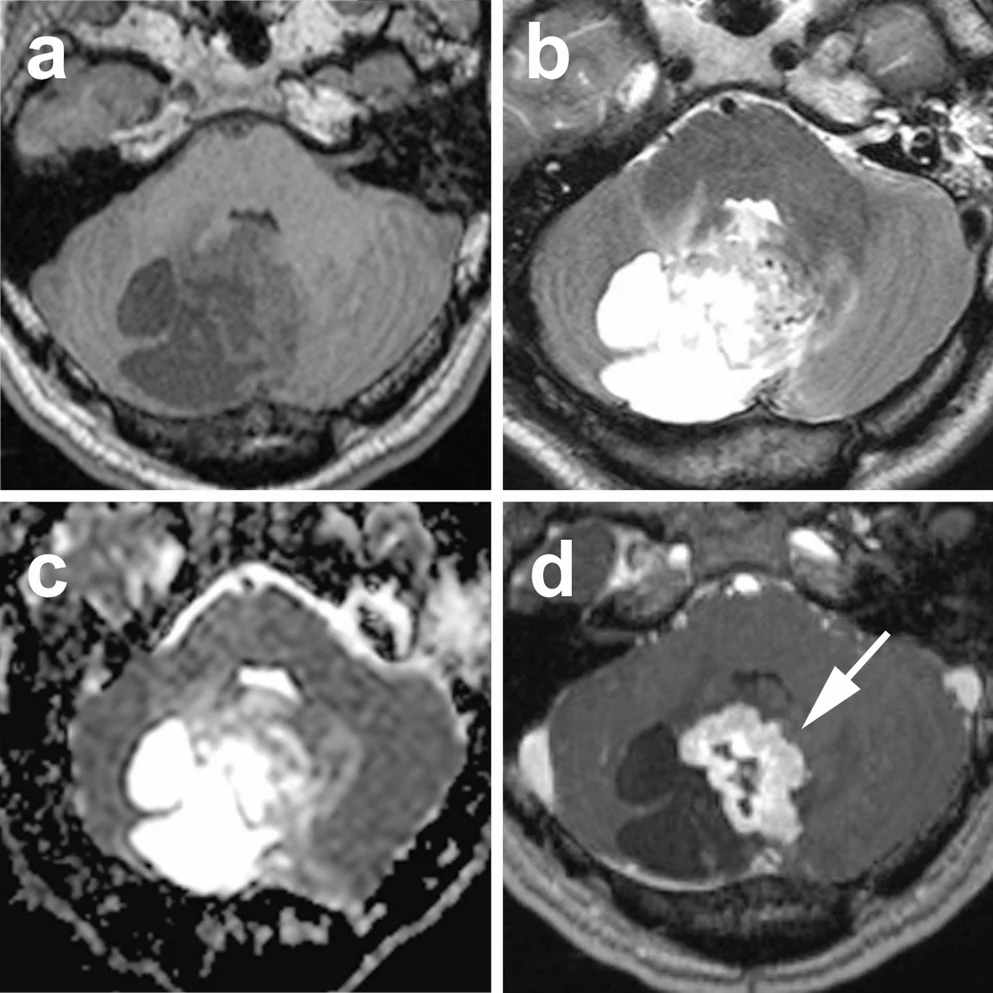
MR images of a 56-year-old man with hemangioblastoma presenting with visual disturbance and headache. Axial T1-weighted image (a), T2-weighted image (b), apparent diffusion coefficient map (c), and contrast-enhanced T1-weighted image (d). The lesion demonstrates a multilocular cystic mass with a relatively large, irregular solid component. The solid portion shows high signal intensity on T2-weighted images with flow voids, indicating prominent vascularity, and exhibits marked enhancement after contrast administration (arrow) without evidence of restricted diffusion
